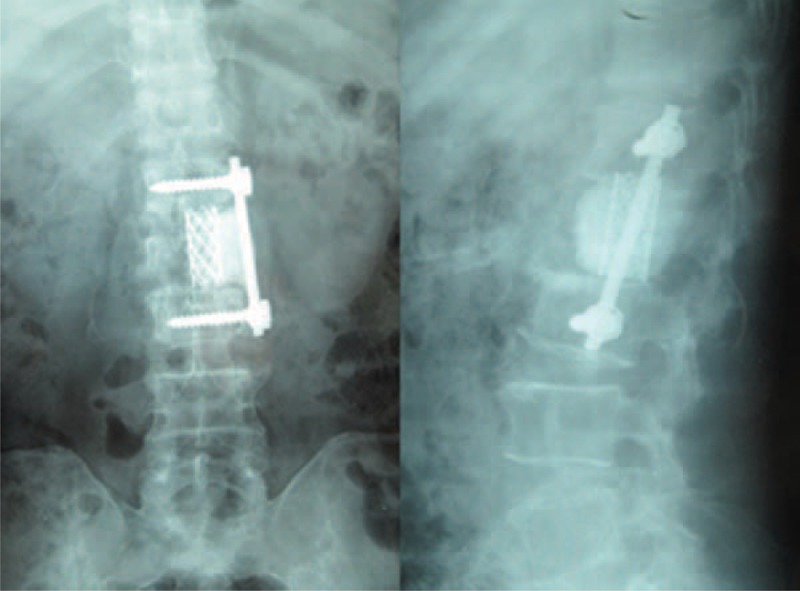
Postoperative radiography. The vertebra was fixed with titanium mesh and a single pedicle screw and was padded with bone cement.
